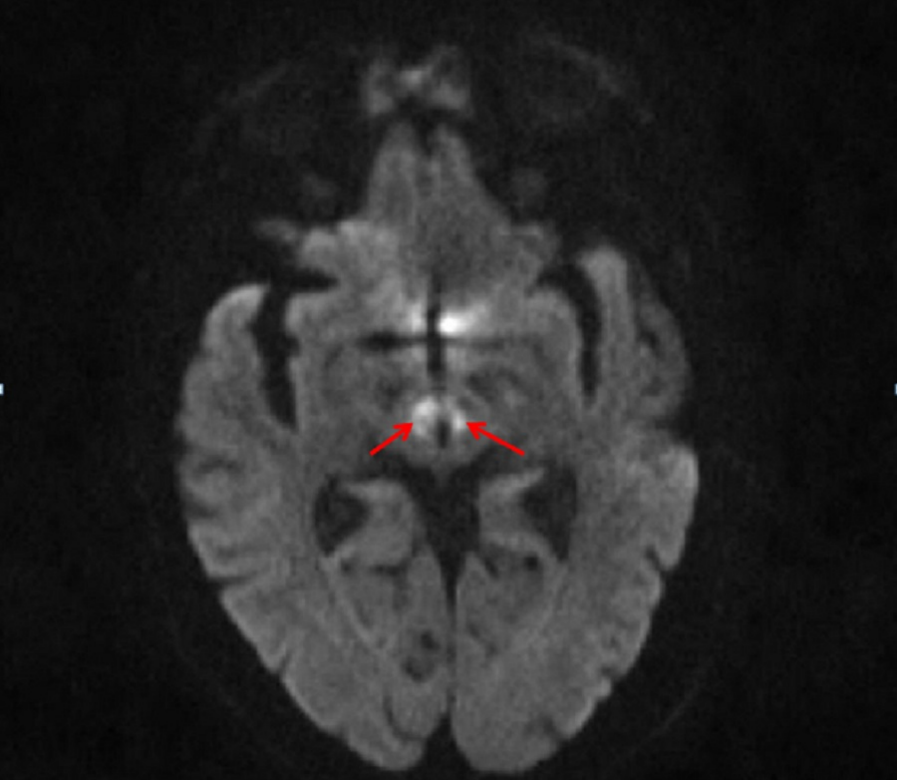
Magnetic resonance imaging (MRI) demonstrating stroke extension into subthalamic nucleus region Axial diffusion-weighted imaging (DWI) sequence showing extension of the stroke into the subthalamic region on both sides (red arrows)
